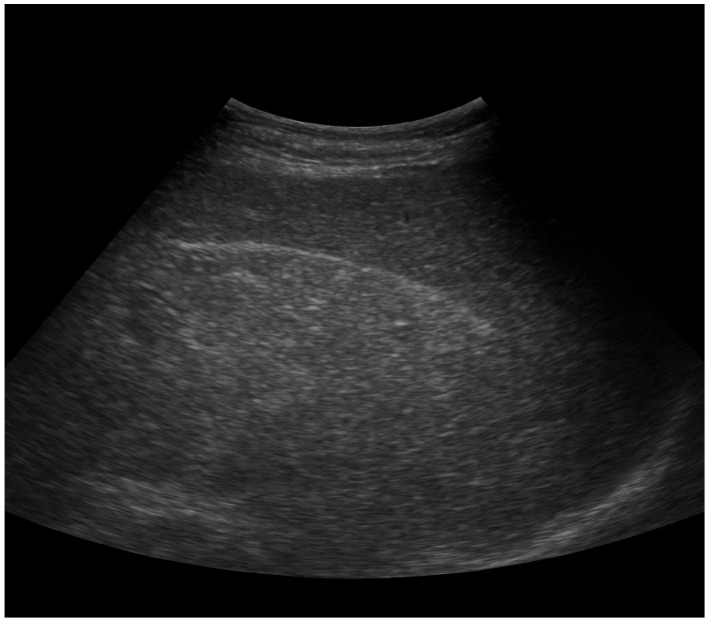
Abdominal ultrasound showing increased liver echogenicity consistent with hepatic steatosis (fatty infiltration).
